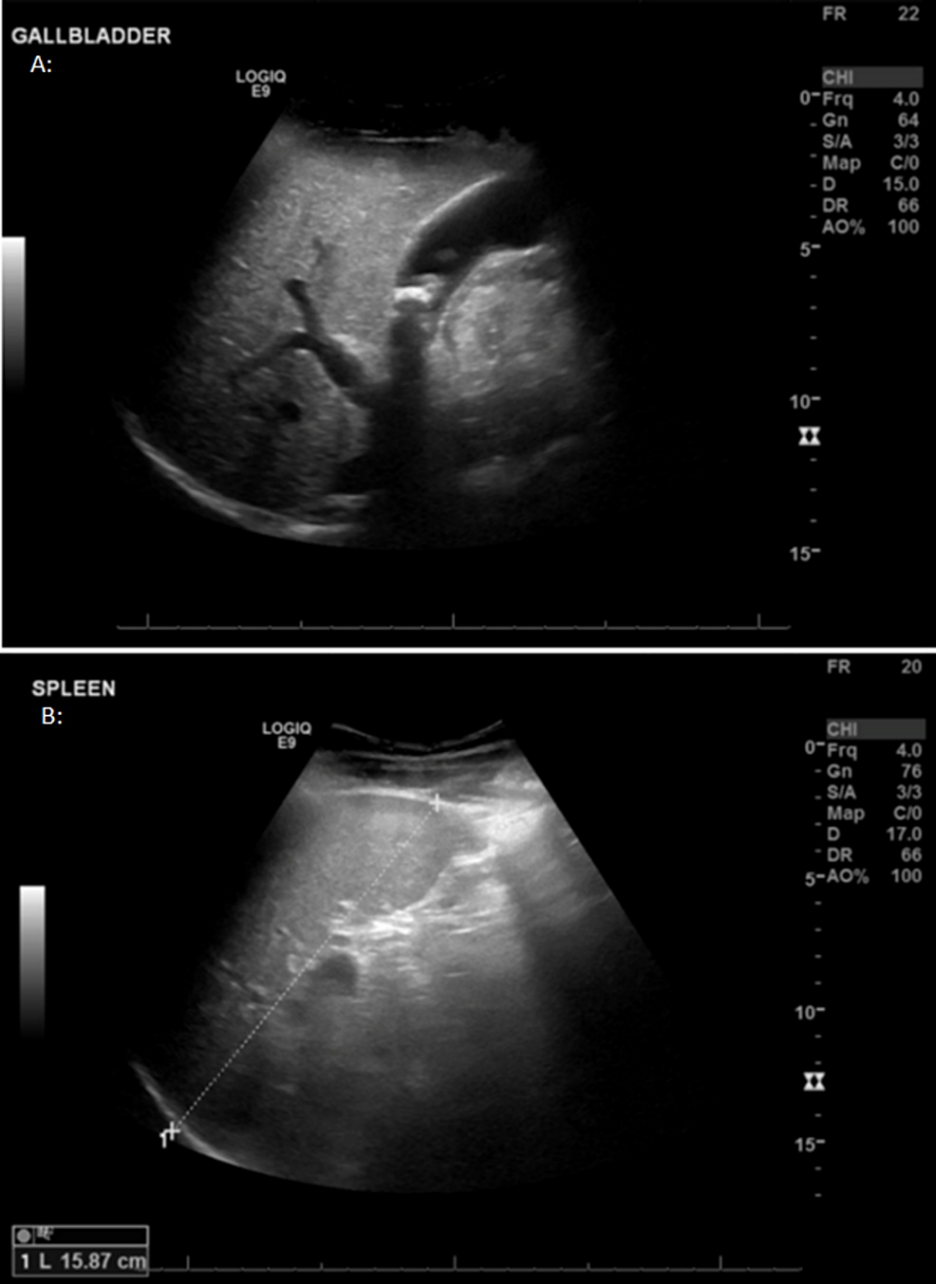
Abdominal ultrasound showing multiple gall bladder stones (A) and splenic enlargement measuring 15.9 cm (B).
